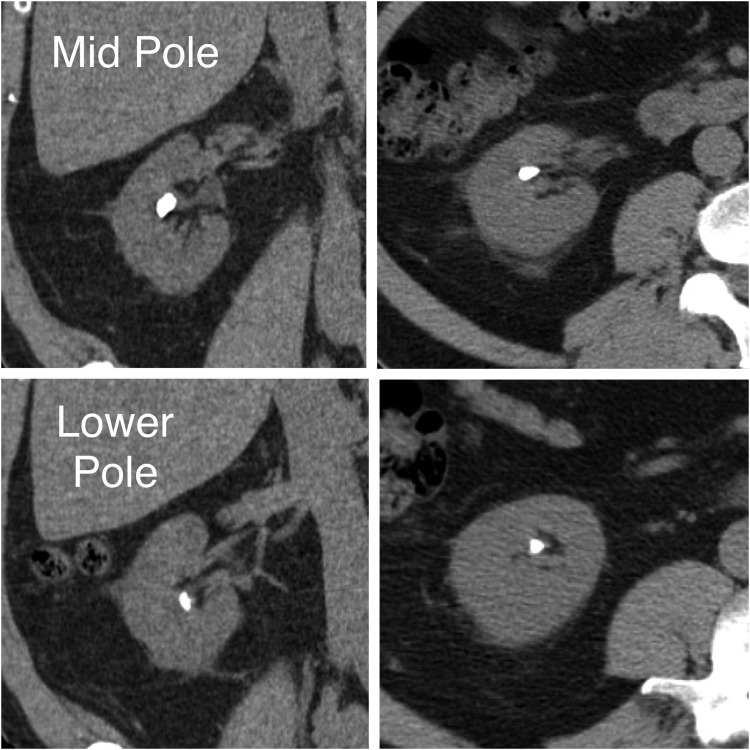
CT demonstrating patient's intra-abdominal anatomy and stone burden.
